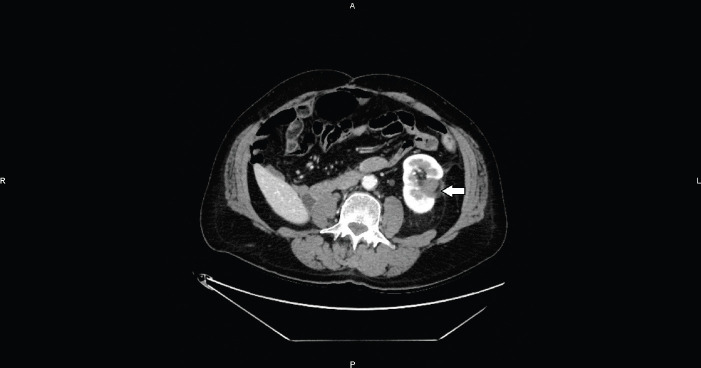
Axial CT image shows the hypo-enhancing ablation zone without enhancing residual tumor (arrow).
